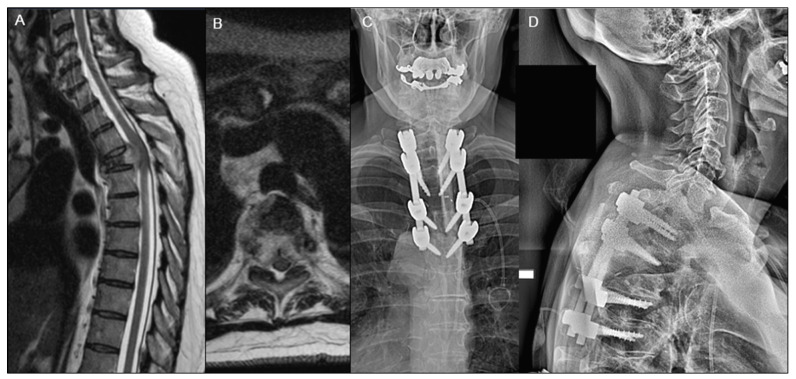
A 61-year-old female patient presented to the emergency department with bilateral leg weakness and right shoulder pain and was found to have Bilsky 3 compression at T4 as seen on preoperative T2-weighted MRI (A,B). The patient underwent separation surgery with thoracic laminectomy, partial of T3, total of T4, with transpedicular corpectomy bilaterally of the T4 vertebral body with greater than 50% removal of the T4 vertebral body and decompression of the spinal cord as seen on her postero-anterior and lateral X-rays (C,D). Despite initial improvement, the patient died a year later from progression of her renal cell carcinoma.
